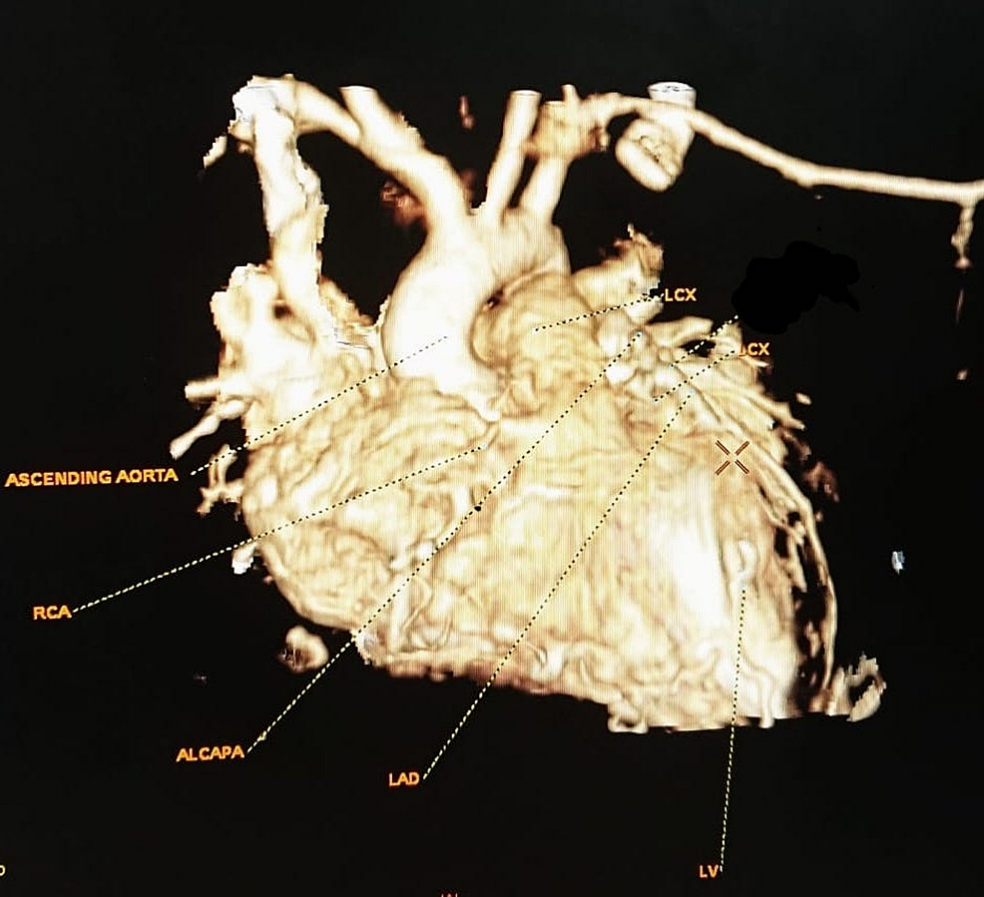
Preoperative Cardiac CT Virtual Reality Scan Showing ALCAPA LCX, left circumflex artery; RCA, right coronary artery; ALCAPA, anomalous left coronary artery from pulmonary artery; LAD, left anterior descending; LV, left ventricle.
